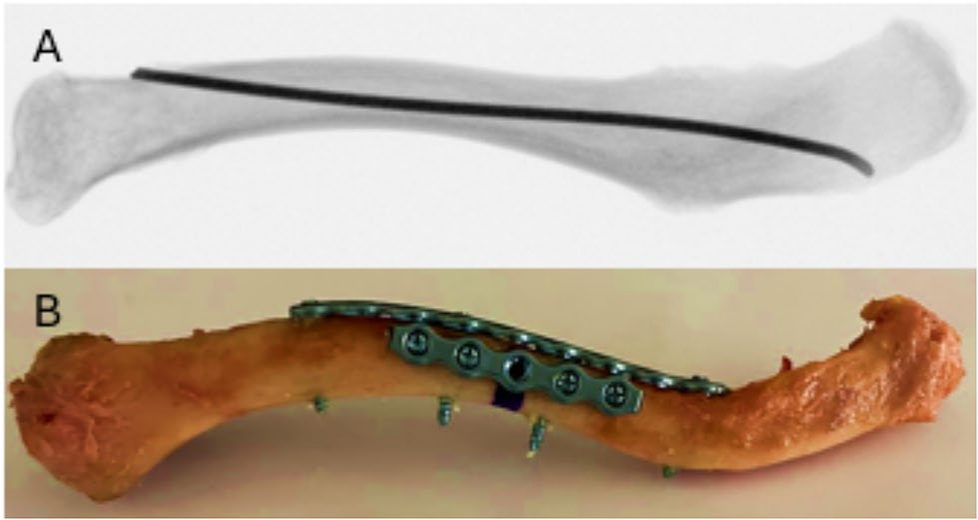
X-ray of a left clavicle instrumented with TEN A) and photograph of a left clavicle instrumented via 2 × 2.0 mm dual plating with the planned osteotomy marked with black ink (B)
